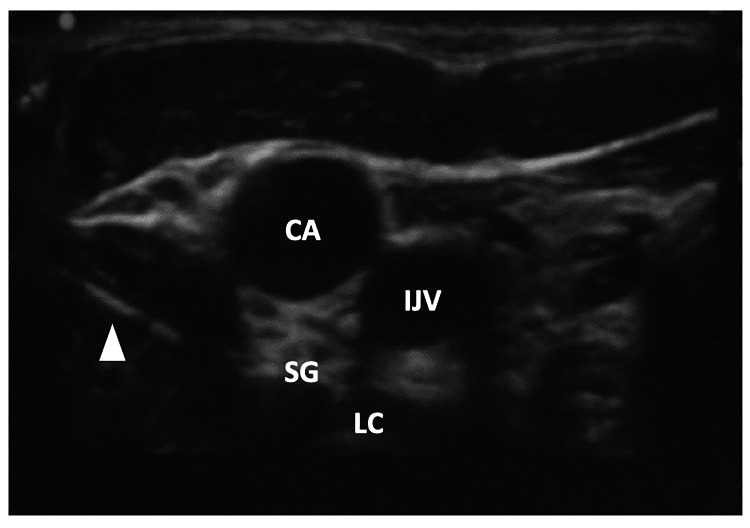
Ultrasound image shows key anatomical structures during stellate ganglion block (SGB). The needle is indicated by the arrowhead. CA: carotid artery; IJV: internal jugular vein; SG: stellate ganglion; LC: longus colli muscle
